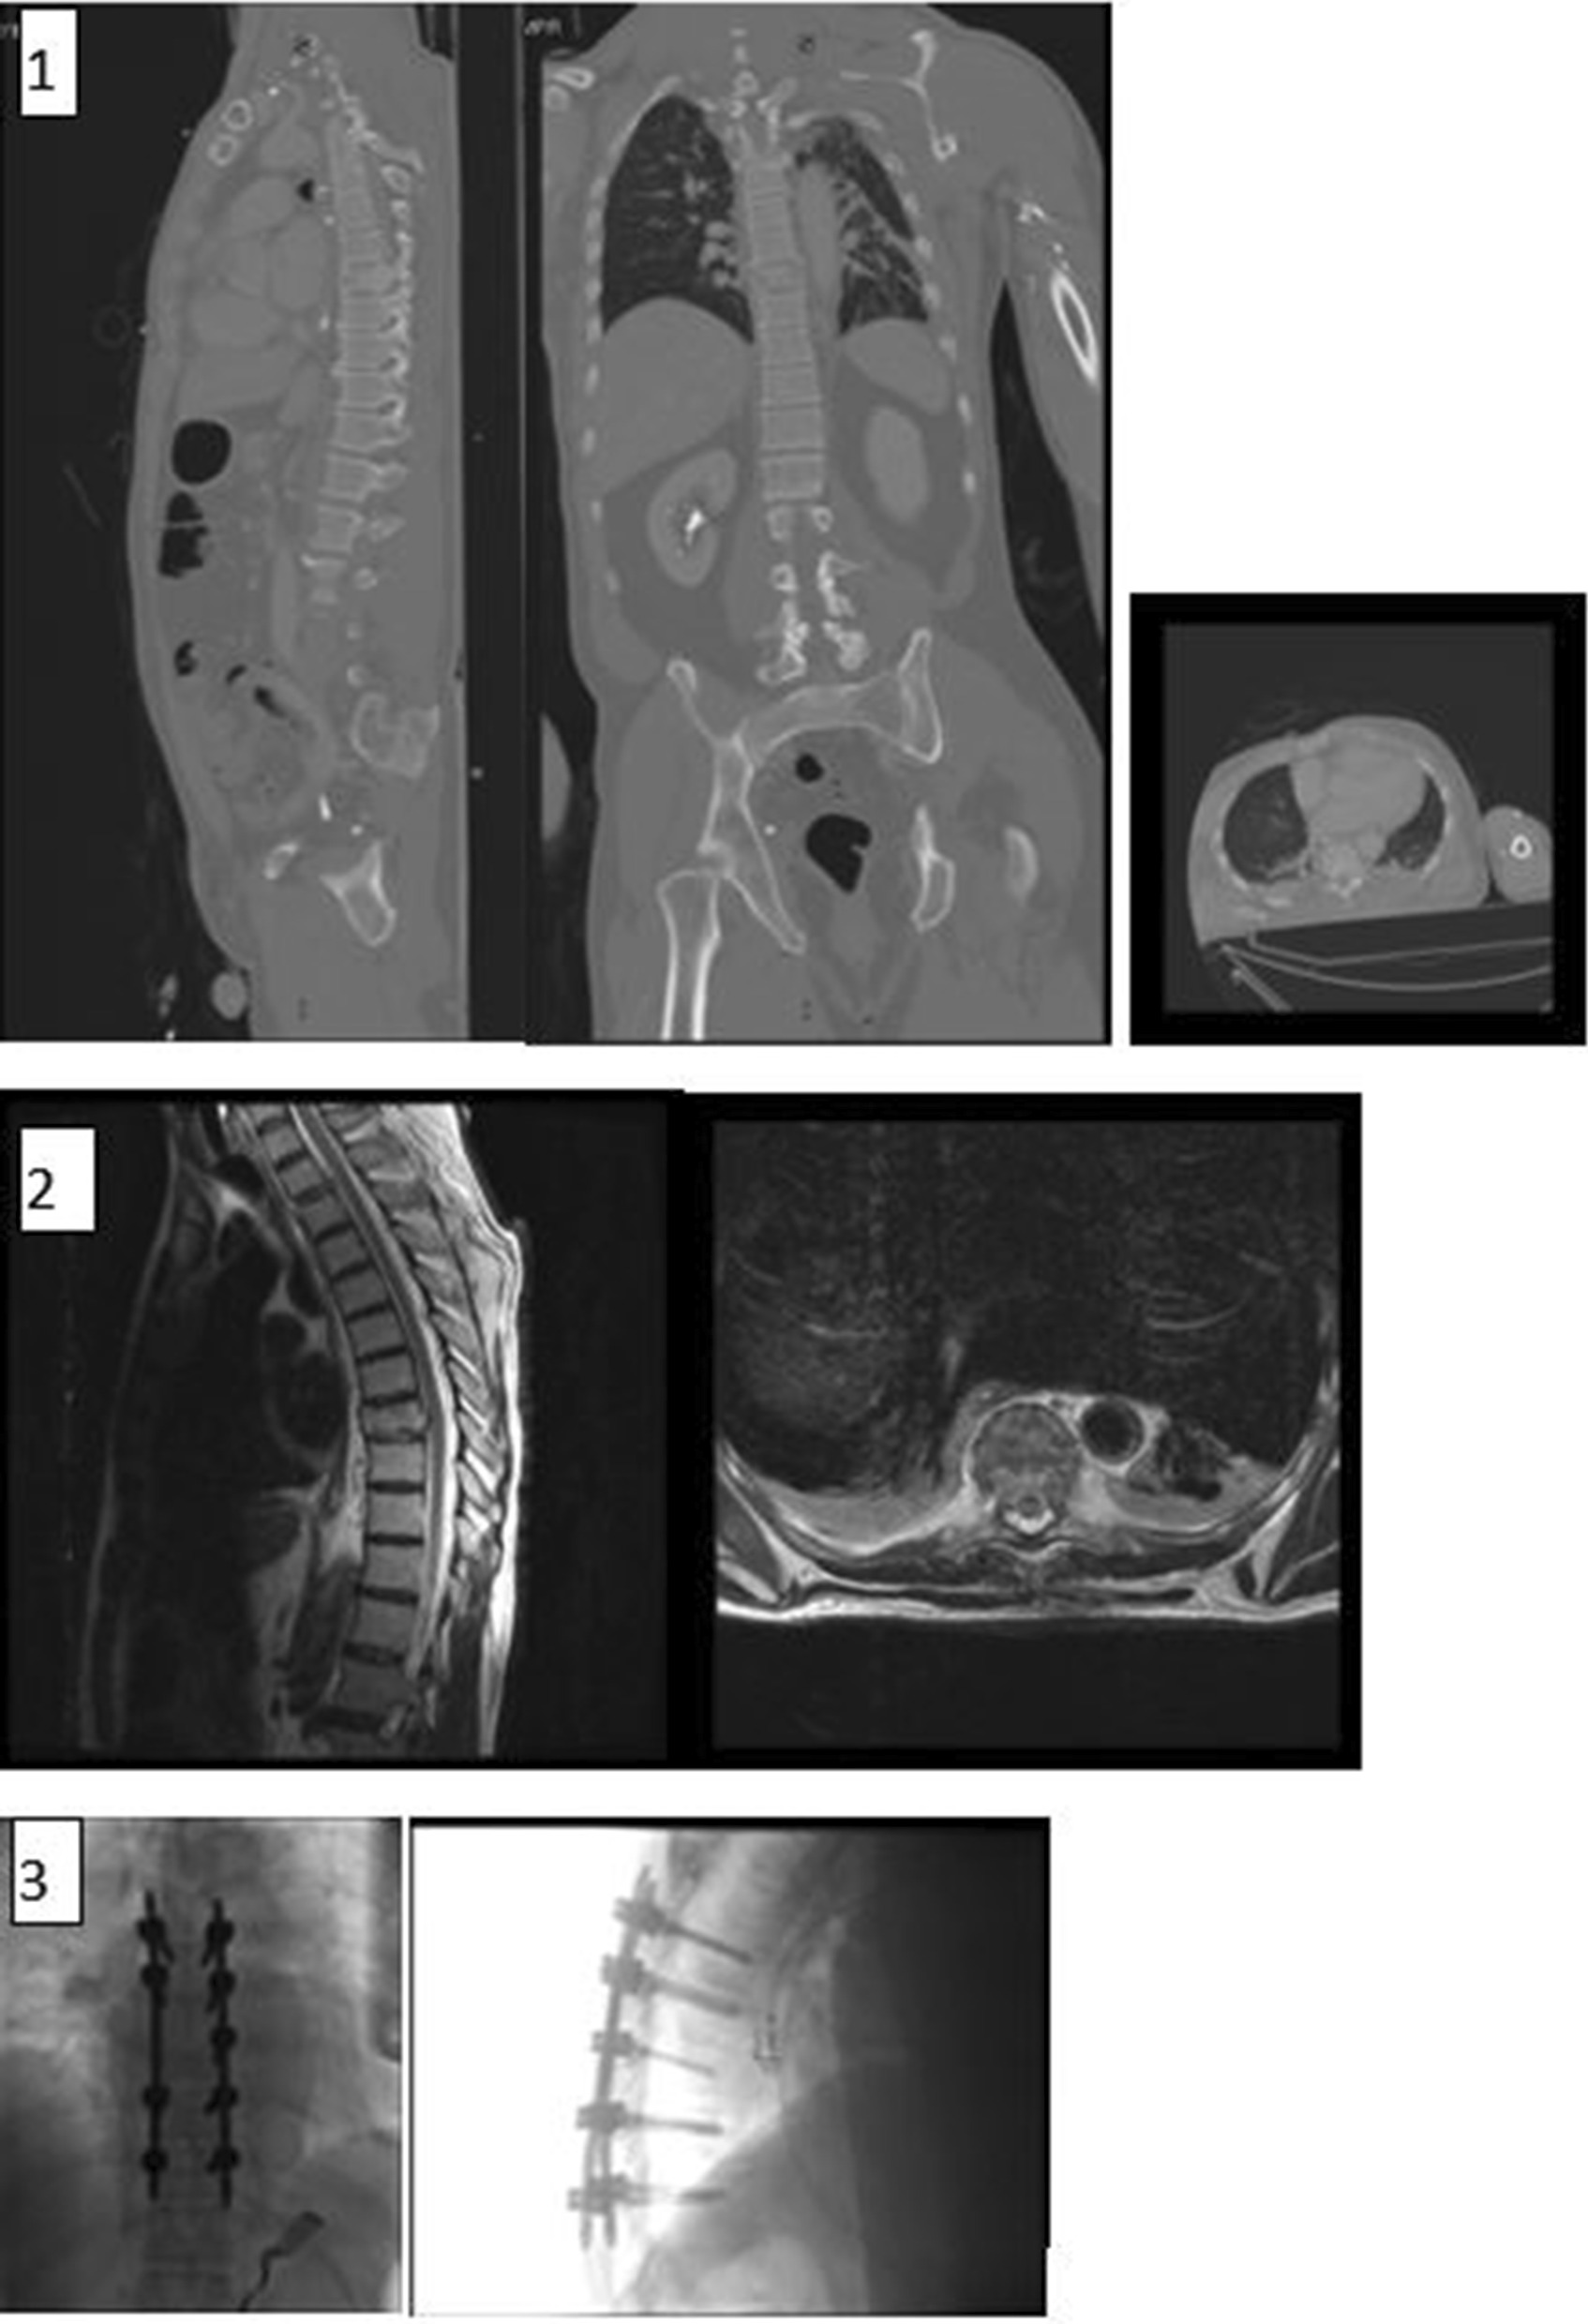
Case 1: 65 Y/O male was admitted with (1) T8 burst fracture due to electrical bicycle accident. (2) The MRI showed no cord injury. (3) The patient had a percutaneous fixation T6-T10 using robotic guidance and 3D intraoperative imaging. The patient had no complications
